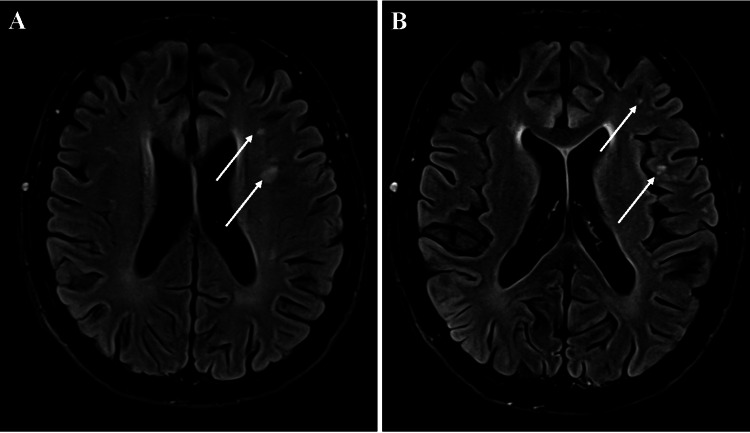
Axial T1-weighted FLAIR MRI of the brain at the level of the lateral ventricles. (A) Superior view. (B) Inferior view. Nonspecific, patchy white matter lesions are highlighted by white arrows and may be secondary to inflammation, infection, trauma, or other causes, including demyelination. Imaging parameters:
